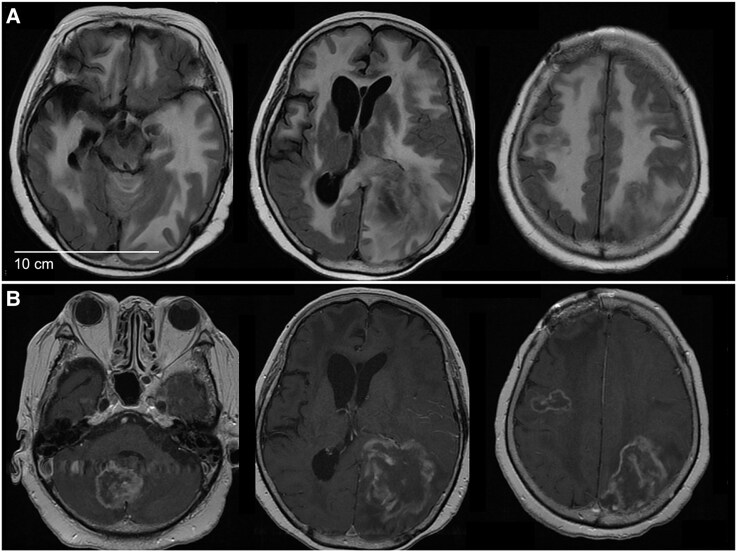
Brain MRI of PT-2 with archaeal infection in advanced stage. (A) DWI shows tumour-like lesions in the white matter of the right frontal and left parietal lobes with high signal intensity in the periphery and low signal intensity in the centre; FLAIR images reveal diffuse hyper-intensity in the white matter and a vague low-signal lesion with midline shift in the left parietal lobe. (B) Ce-T1WIs show enhancement at the margins of the lesion, consistent with the lesion seen on DWI. DWI, diffusion-weighted imaging; FLAIR, fluid attenuated inversion recovery; Ce-T1WIs, contrast-enhanced T1-weighted images.
